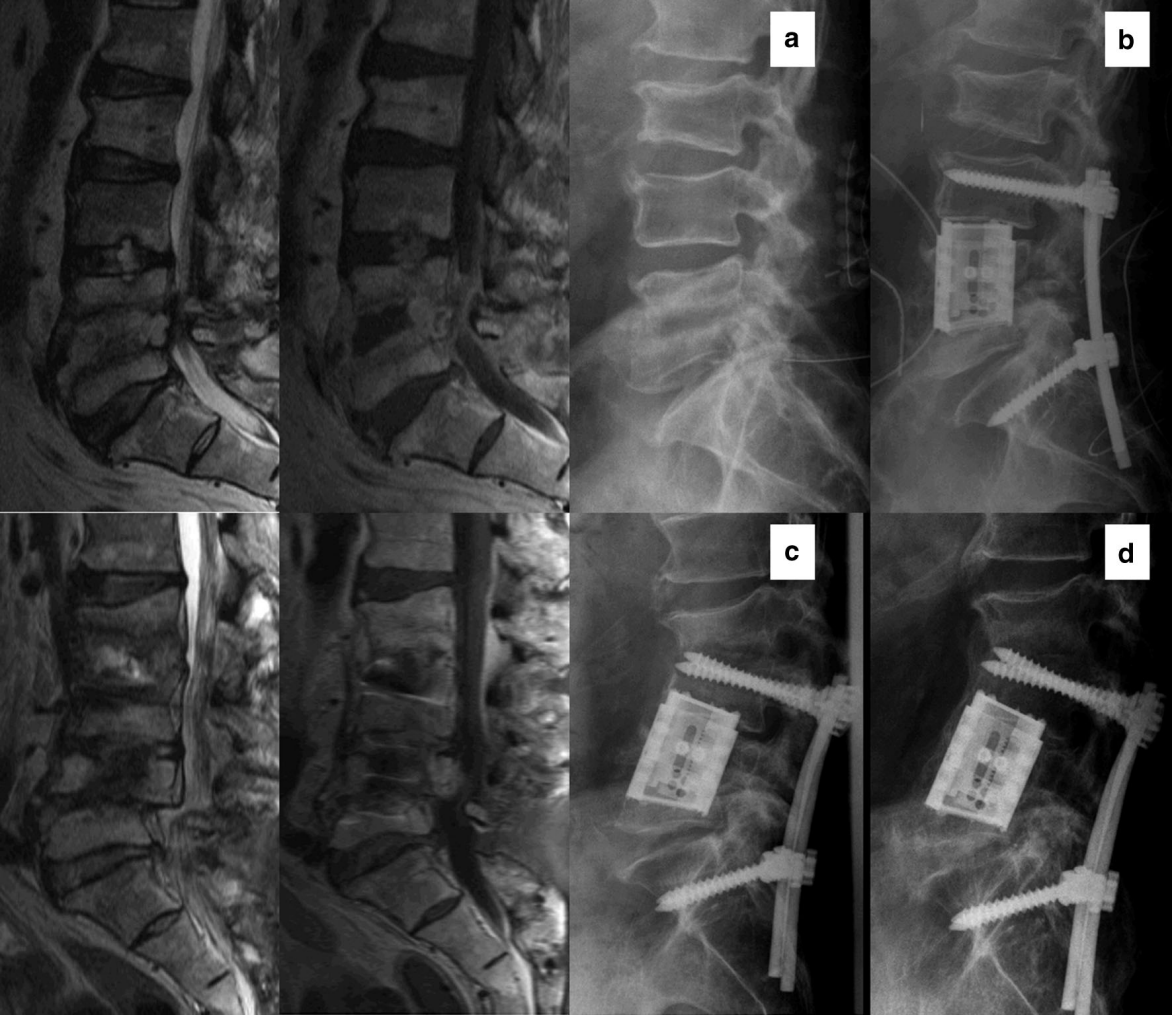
Patient 4: sagittal MRI cuts T2- and T1-weighted, and lateral radiographs; a preoperatively, b after primary operation, c adjacent segment infection, screw loosening and marked adjacent segment kyphosis and d last FU after 5.5 years
